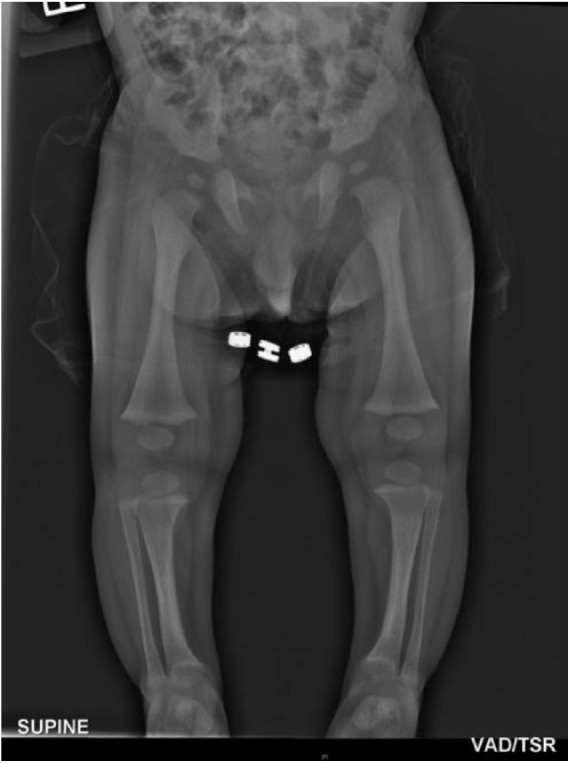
Long limb radiograph showing the features of the lower limbs of the 7-month-old infant. The long bones of the lower limbs demonstrate splaying of the metaphyses with fraying, but no widening of the epiphysis. There is also mild bowing of the tibia.
